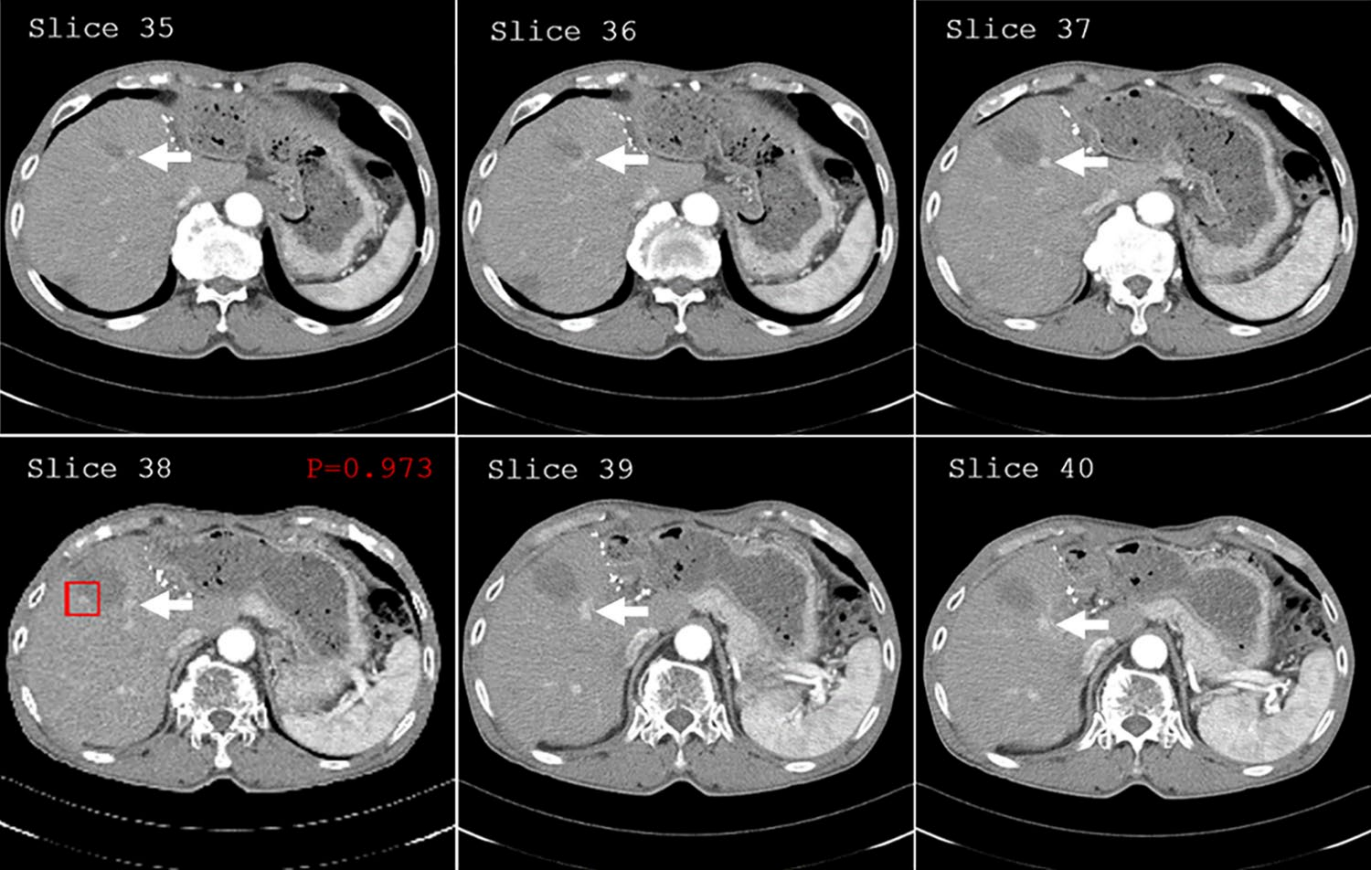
Prediction map for true positive predicted LTP region. In the prediction map, we report the rectangular region of interest on the axial slice of the whole CT scan, slide number, tumor prediction probability, as well as neighboring image slices included in the positive-predicted regions. LTP was detected (red rectangular), and hepatic vessels including hepatic artery and portal vein (arrows) were not indicated in the prediction map.
